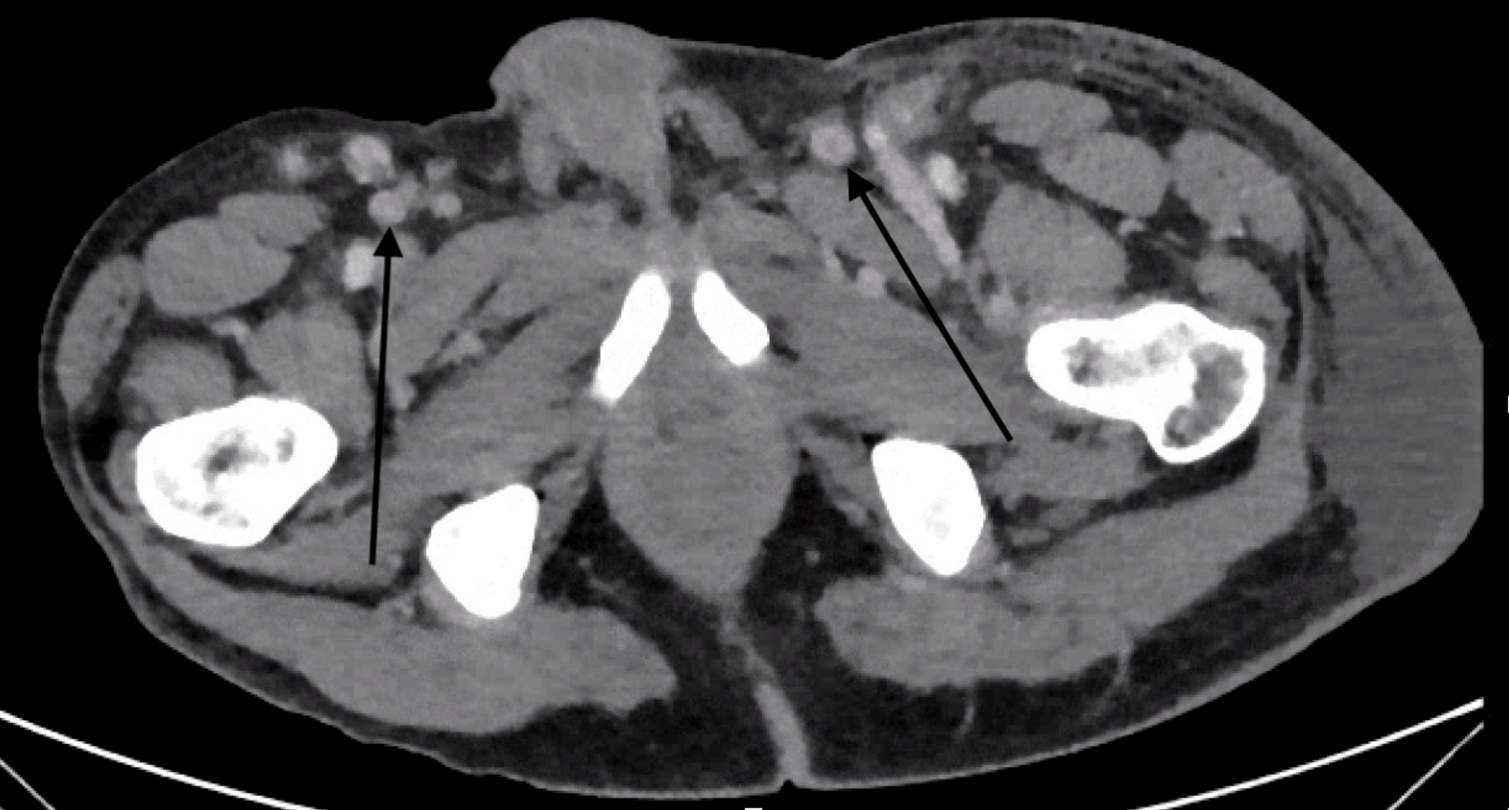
Computed tomography scan of the abdomen and pelvis showing inguinal lymphadenopathy
